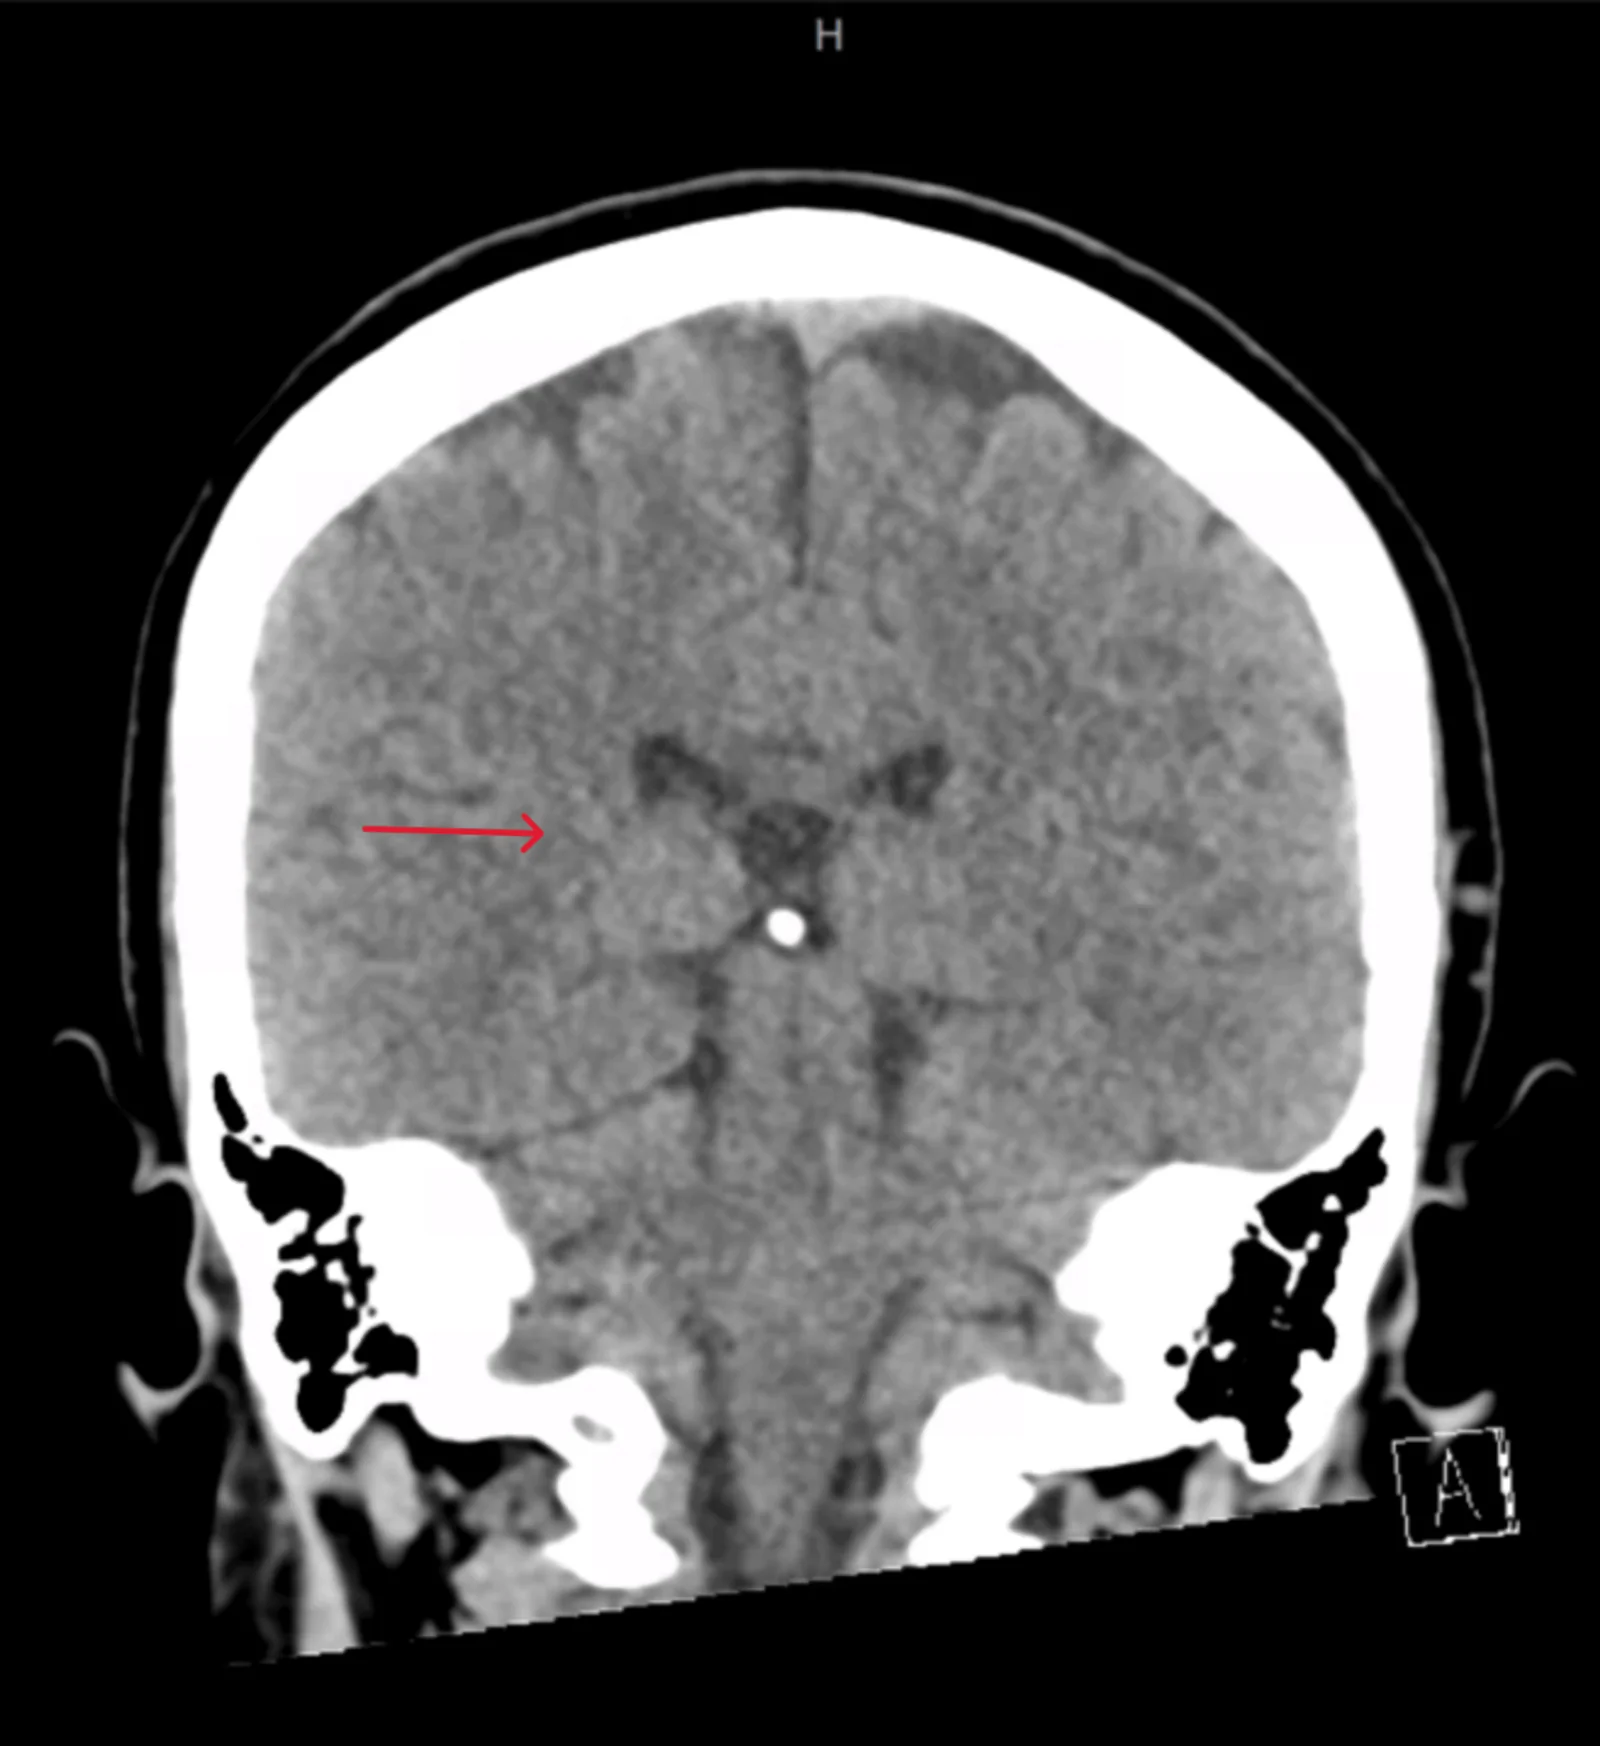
Non-contrast computed tomography (CT) of the head demonstrating no acute intracranial pathology The straight arrow indicates a normal anatomical landmark for orientation.
